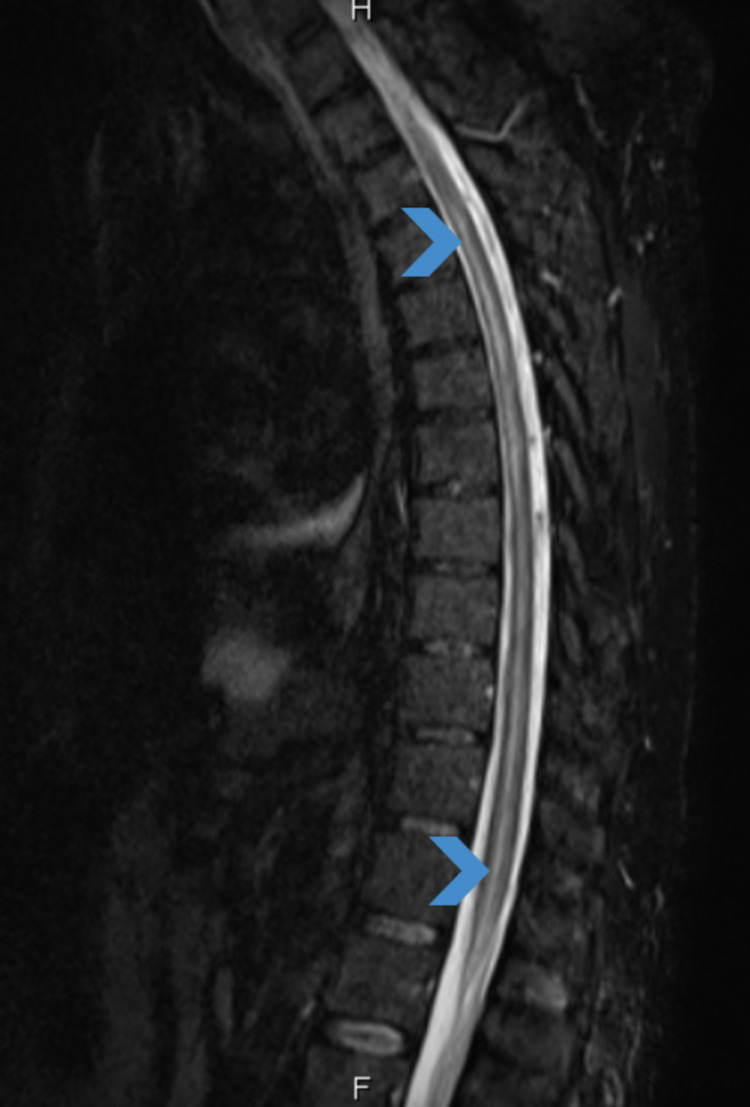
Sagittal short tau inversion recovery MRI of the dorsal spine pretreatment. The MRI demonstrates long segment intramedullary abnormal high signal intensity. MRI: magnetic resonance imaging
